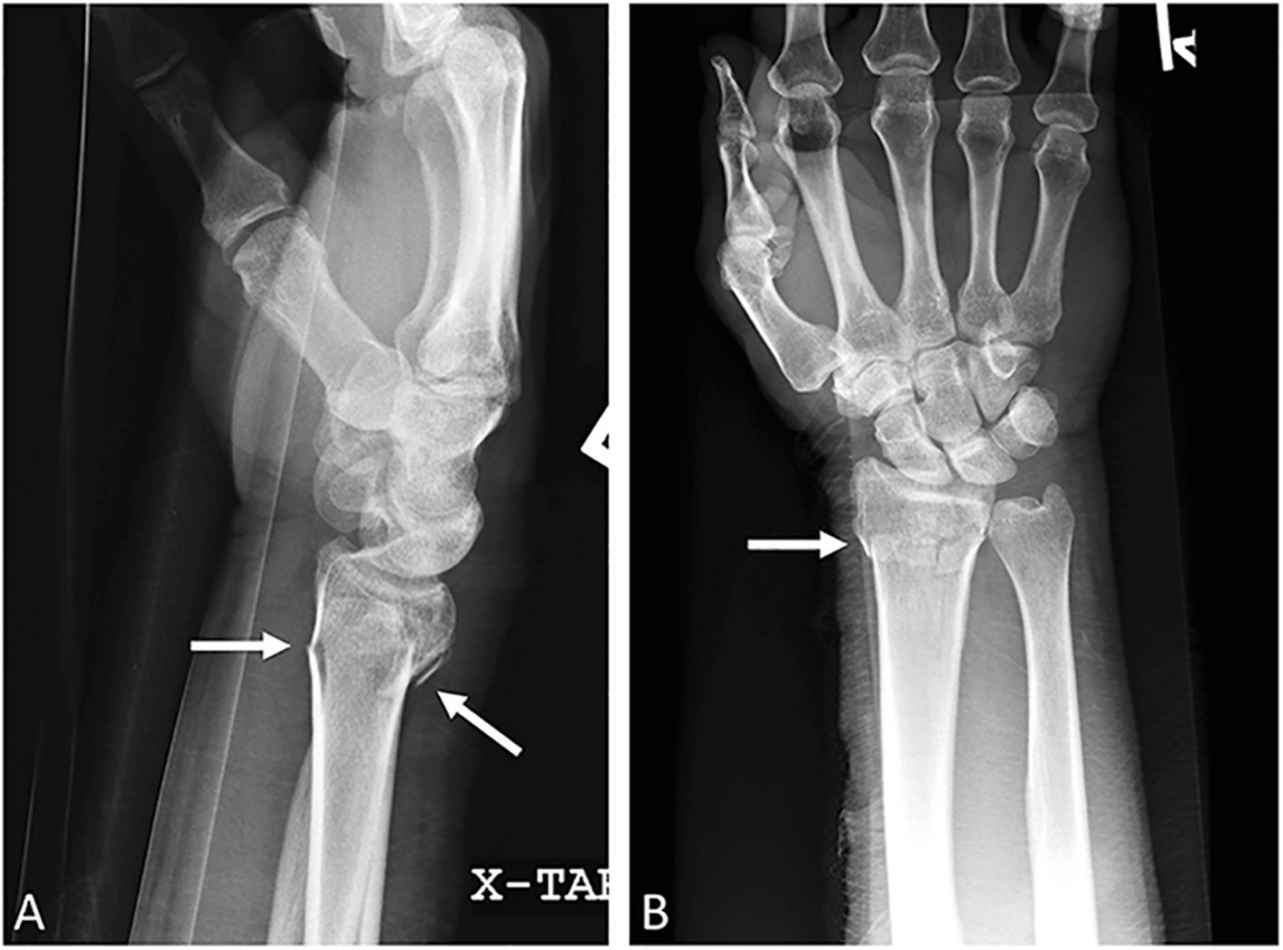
Colles fracture. Lateral (a) and AP (b) wrist radiographs. Transverse distal radial metaphyseal fracture (*arrows*) with no intra-articular extension. Mild dorsal angulation of the distal with respect to proximal fracture fragment; it is this angulation that differentiates the Colles fracture from the Smith fracture, which has volar angulation or displacement
